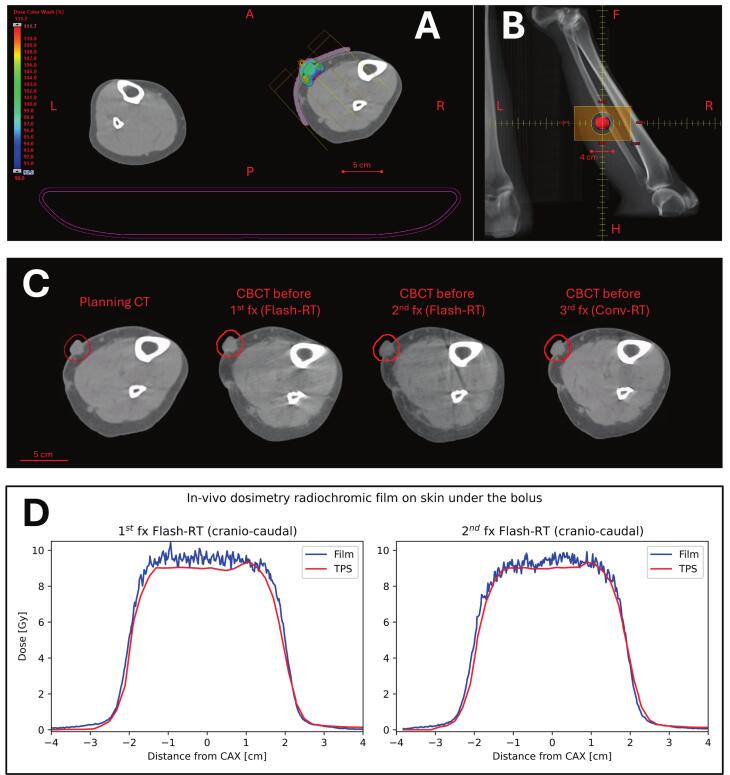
Patient positioning and dosimetry. A: Axial view of the treated planning target volume (PTV, red); B: Beam's eye view; C: Image-guidance for each fraction implementing cone-beam computed-tomography; D: Dosimetric results for each e-FLASH fraction confirming correct dose application for the same patient; Abbreviations: RT − Radiotherapy, CONV – Conventional, CT – Computed-tomography, FX – Fraction of Radiotherapy. (For interpretation of the references to colour in this figure legend, the reader is referred to the web version of this article.)
